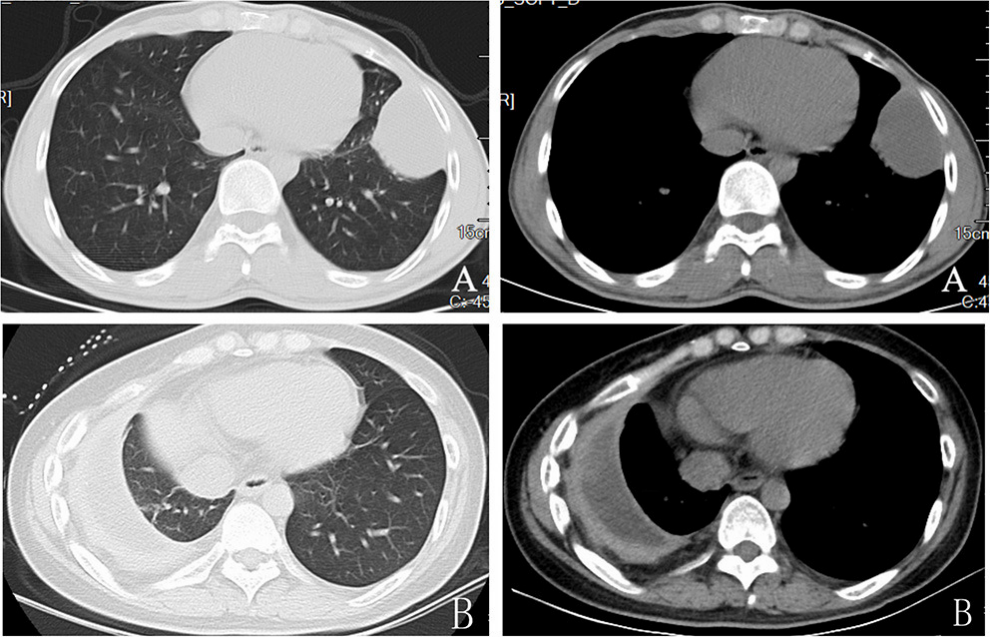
Preoperative computed tomographic scan showing thickened parietal and visceral pleura with collection.Stage II ertuberculosis empyema (A)and stage III tuberculosis empyema (B).
